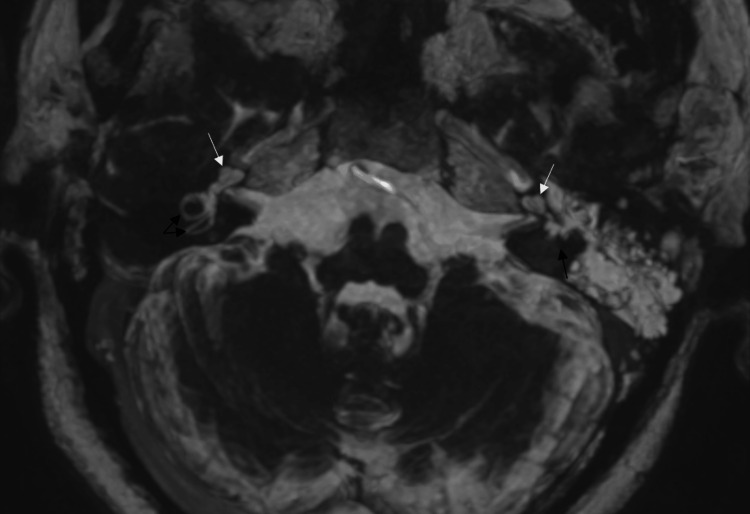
Axial T2-weighted MR images following MIP reconstruction; signal loss from all left three semi-circular canals Black arrows: semi-circular canals; white arrows: cochlea MIP: maximum intensity projection
